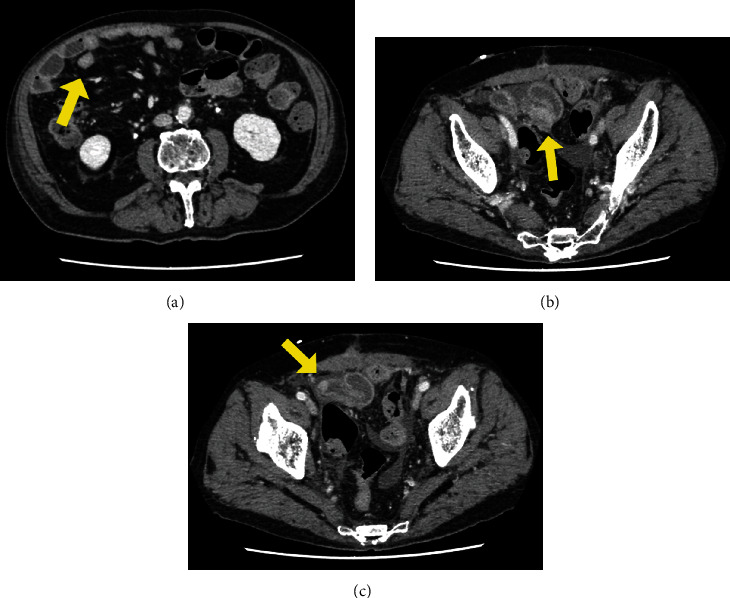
A postoperative contrast-enhanced abdominal computed tomography (CT) scan showed enlargement of one of the peritoneal nodules (a, arrow) and the invasion of nodules with edematous lumens to the small intestine (arrows) (b, c).
